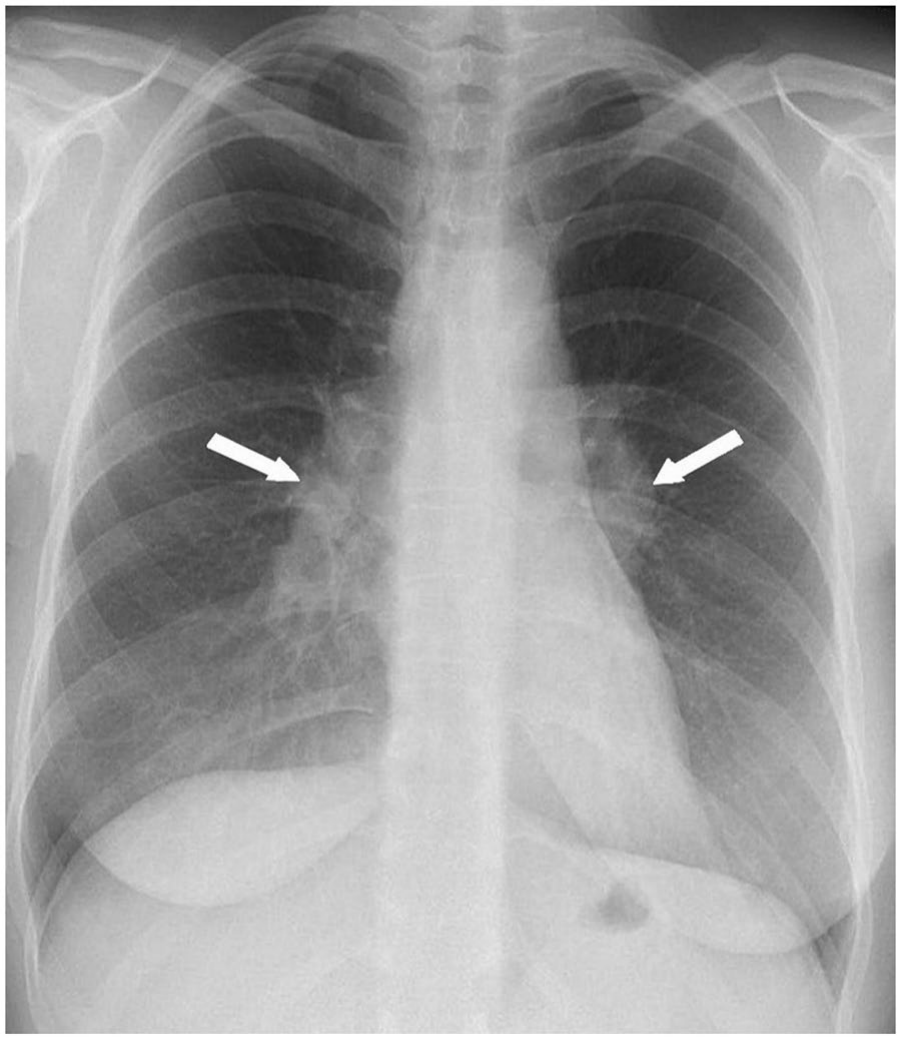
Chest X-ray of patient A showing bilateral lymphadenopathy (arrows).
